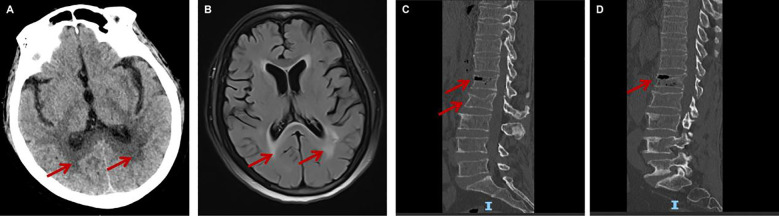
Imaging findings. (A, B) Non-contrast brain CT and MRI scans obtained after admission, showing interstitial cerebral edema and old lacunar infarcts. (C, D) Non-contrast lumbar spine CT obtained after admission, demonstrating bone marrow edema, gas accumulation or osseous destruction at the T12 and L1 vertebrae.
